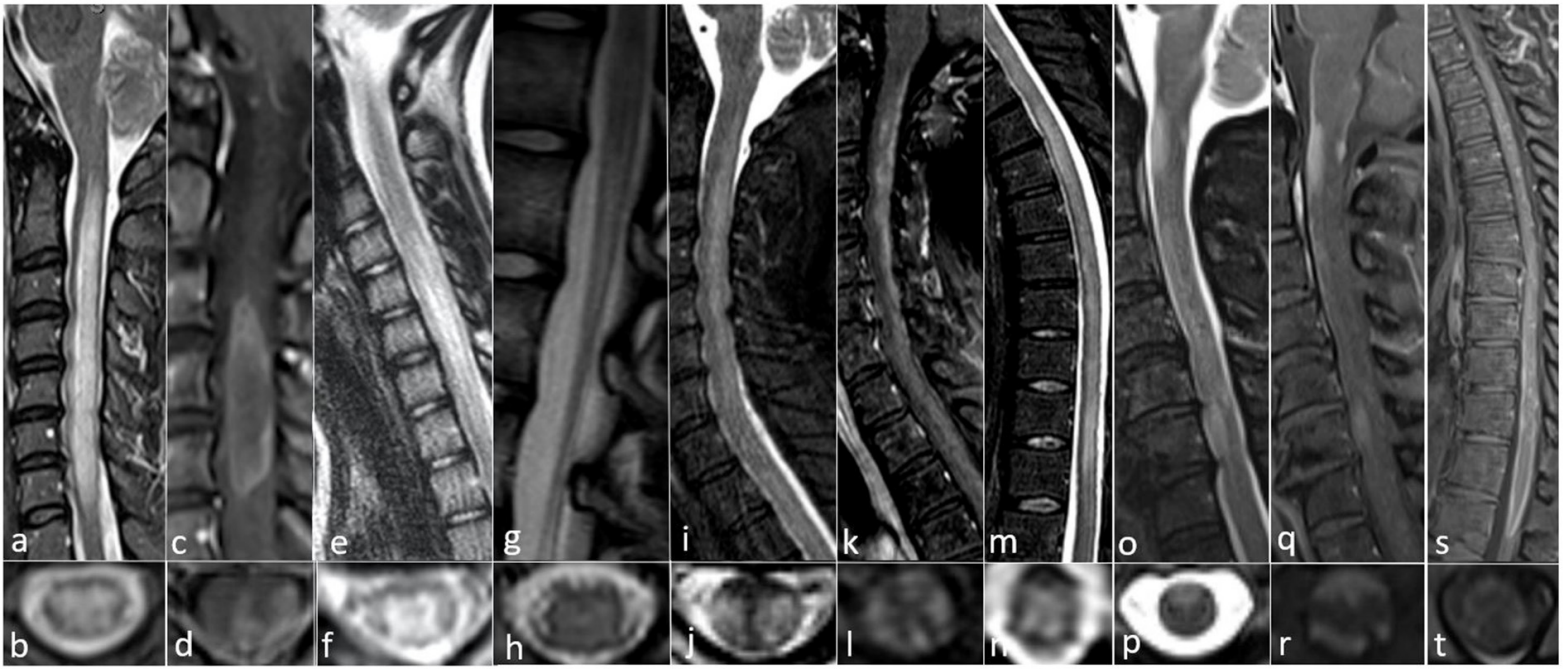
All images collected from cases seen at the Autoimmune and Neuroimmunology clinics at the University of Colorado. (a–d) AQP4-IgG positive 52-year-old woman with progressive limb weakness and sensory loss with MRI showing (a) sagittal confluent T2 hyperintensity with cord edema from C2 to T1, (b) axial central predominant T2 hyperintense lesion, (c) sagittal ring-enhancement pattern from C3 to C5, and (d) Axial ring enhancement pattern with central and lateral involvement. (e–h) MOG IgG positive 12-year-old-girl with gait abnormality and mild lower extremity weakness with MRI showing (e) sagittal T2 hyperintensity with mild cord edema from C4 to C7, (f) axial gray matter T2 hyperintensity with appearance of an “H” sign, (g) sagittal T2 hyperintensity of the conus medullaris, and (h) Axial subtle symmetric central T2 hyperintensity. (i–n) CRMP5 IgG positive 54-year-old woman with paraplegia, found to have a neuroendocrine carcinoma suggestive of thymus or small cell lung origin and MRI with (i) sagittal T2 hyperintensity with cord swelling from C2 to T1, (j) axial posterior and lateral T2 hyperintensity, (k) sagittal dorsal subpial contrast enhancement from C2 through T1, (l) axial anterior, lateral, and dorsal subpial contrast enhancement, (m) sagittal T2 hyperintensity from T1 to T11, and (n) axial central predominant T2 hyperintensity. (o–t) GFAP IgG positive 63 year old woman with subacute progressive encephalomyelitis with no underlying malignancy and MRI findings of (o) sagittal patchy, extensive ventral T2 hyperintense lesions from the craniocervical junction to C7, (p) Axial ventral T2 hyperintensity, (q) sagittal dorsal and central enhancement at the margin of the craniocervical junction as well as ventral enhancement at the margin of the cord at C3 and C6, (r) axial dorsal and ventral subpial enhancement, (s) sagittal diffuse enhancement of the ventral and dorsal margins of the distal thoracic cord from T8 to T11, and (t) axial ventral subpial enhancement.
